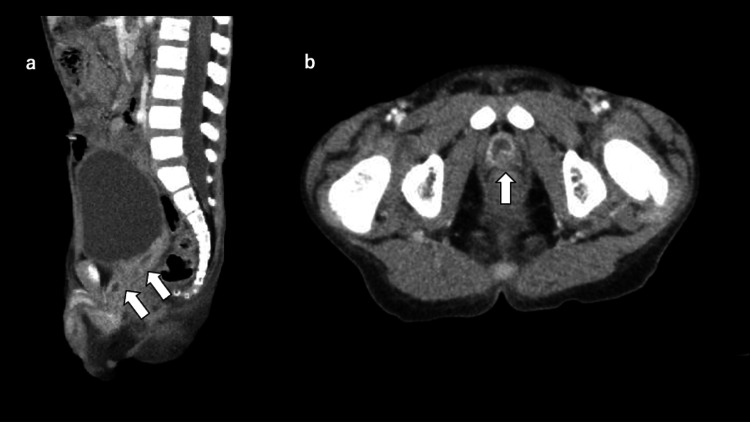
Contrast-enhanced computed tomography A contrast-enhanced computed tomography scan revealed multiple prostatic abscesses with rim enhancing and fluid collection. (a) Sagittal view, (b) axial view.
